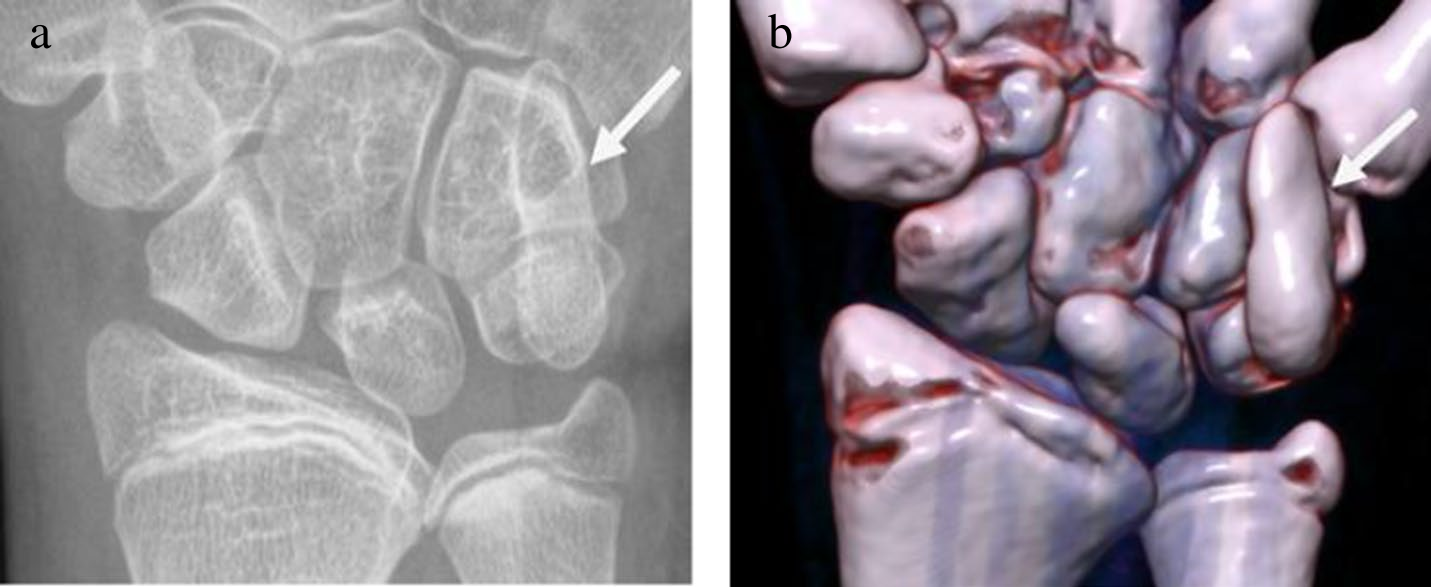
A 16-year-old female with Lennox-Gastaut syndrome was evaluated for asymptomatic right wrist swelling. PA radiograph (a) revealed an enlarged hook of the hamate (arrow). 3D reconstructions of a CT (b) confirmed osseous pisiform-hamate coalition (*arrow*). The hook of the hamate (also known as the hamulus) and pisiform bone form a distinctive connection in osseous pisiform-hamate coalition. The appearance of a “hefty hamate hook” has not been reported previously but may be a sign to help diagnose this condition on radiographs. Pisiform-hamate coalition is uncommon and differs from other wrist coalitions by involving both carpal rows. To our knowledge, it has not been associated with Lennox-Gastaut syndrome. Although this coalition is often asymptomatic, it is more prone to fracture and can be a source of ulnar nerve entrapment.
